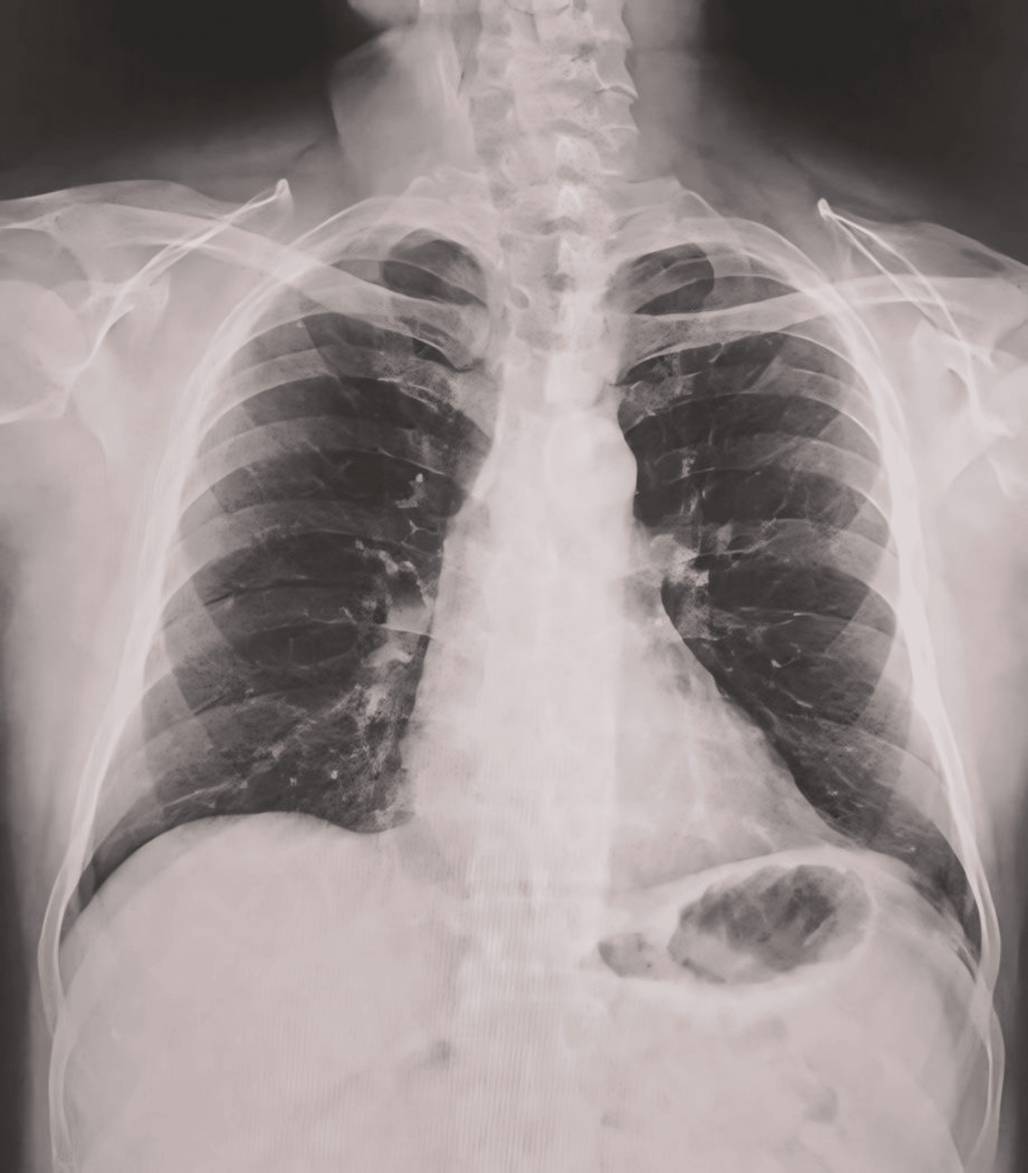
The chest X-ray shows no evidence of pneumothorax or pneumomediastinum.
